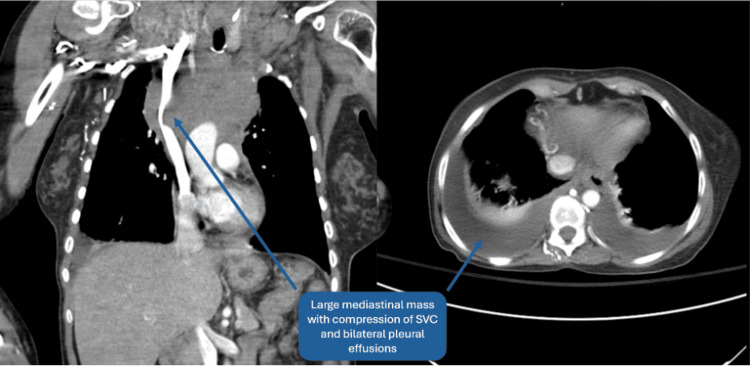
Chest CT with contrast: coronal series demonstrates a large mediastinal mass with compression of the superior vena cava, and axial series demonstrates moderate bilateral pleural effusions. CT: computed tomography
